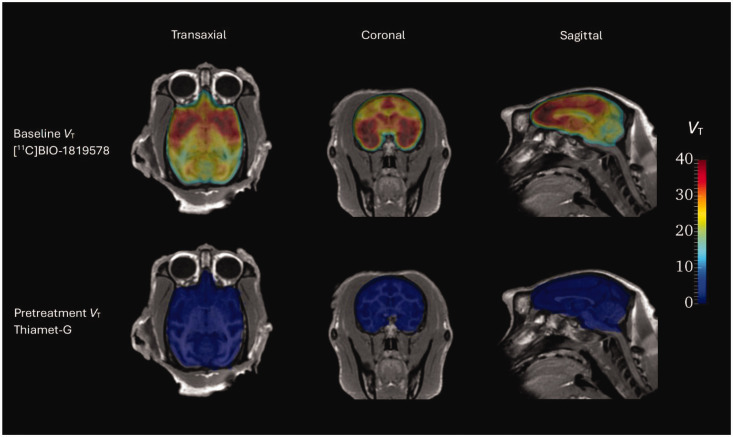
Parametric V_T_ images of [^11^C]BIO-1819578 at baseline and under pretreatment with Thiamet-G. Both sets of data are overlayed on corresponding MR image in NHP3.
